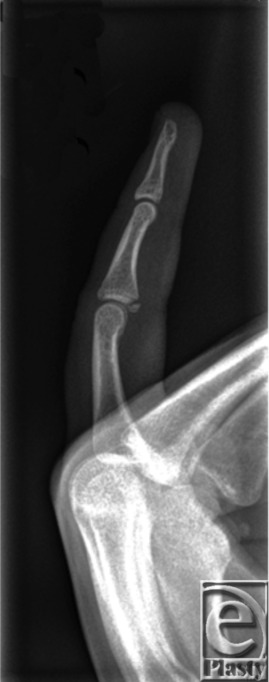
Radiograph demonstrating a volar plate avulsion fracture (Eaton type 3a).
